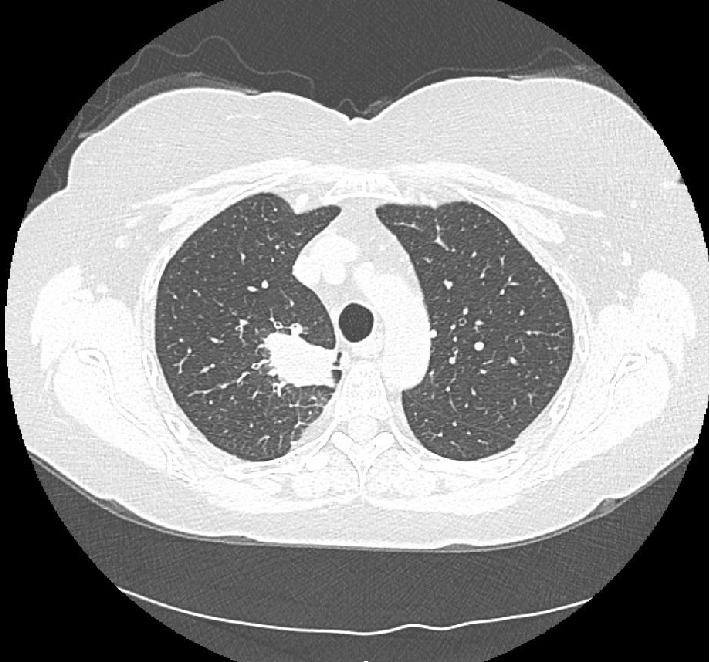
CT scan of the thorax showing a large right upper lobe spiculated nodule.
